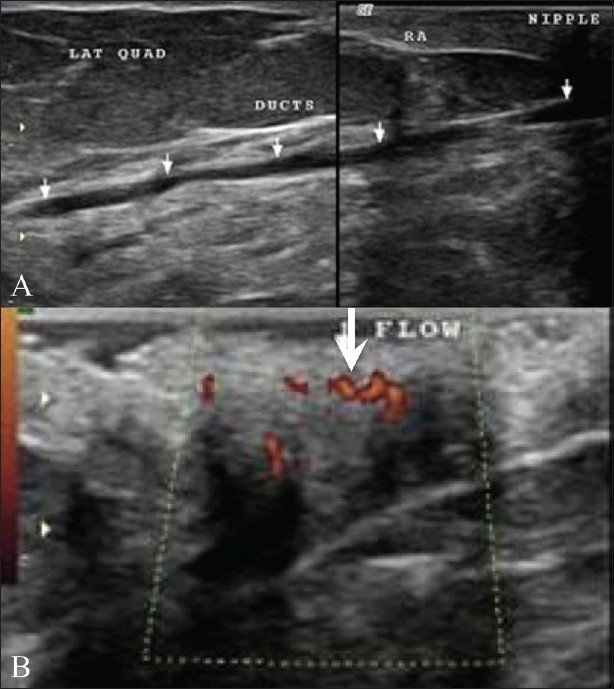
Paget's disease. A 53-year-old lady presented with excoriation of the right nipple and no palpable lump. Mammograms showed no significant findings. Breast USG (A) shows a prominent duct (arrows) in the lateral quadrant. Increased vascularity (arrows) is seen on color Doppler examination (B) in the subareolar region. A clinical diagnosis of Paget's disease was made, which was confirmed on skin biopsy. The patient subsequently underwent a mastectomy
